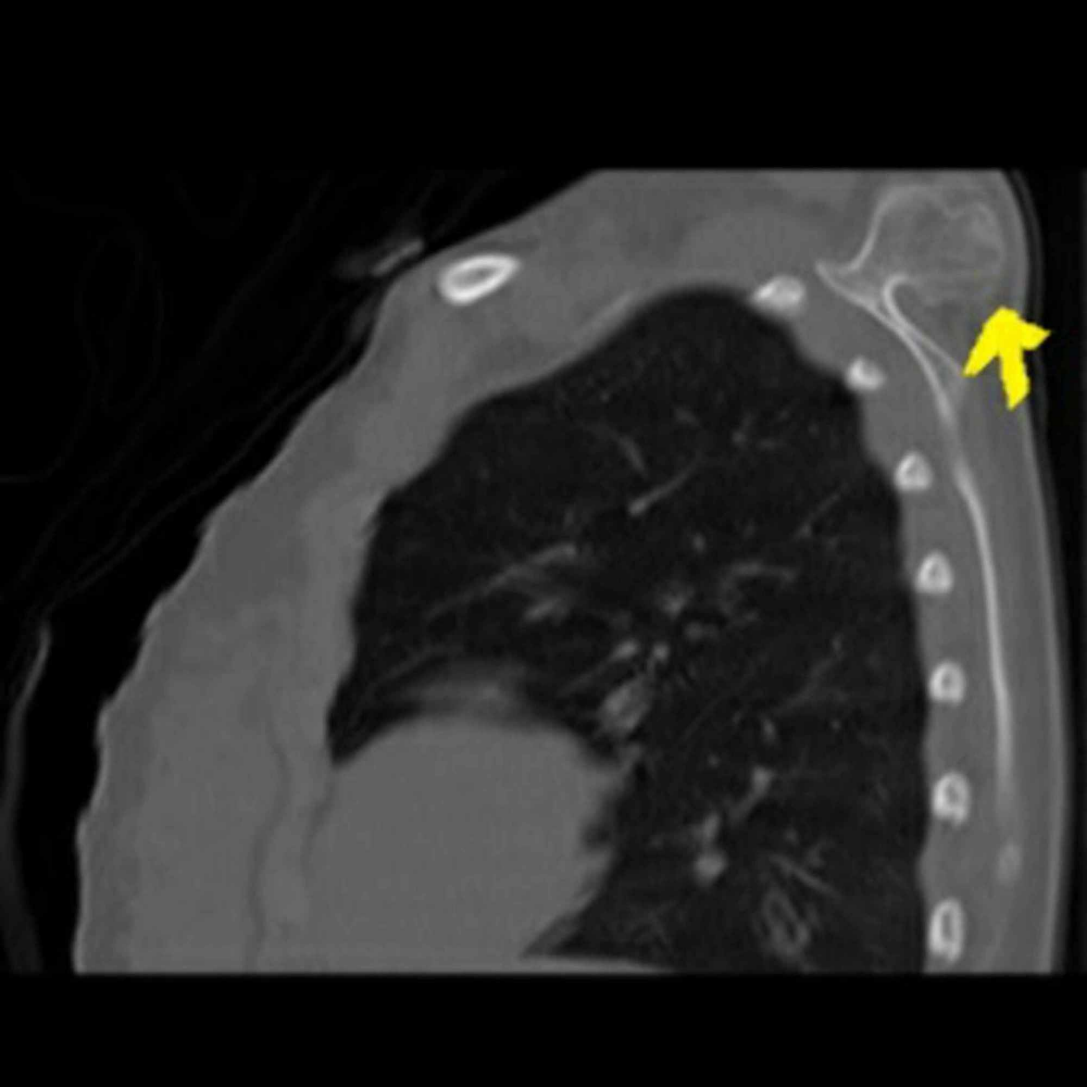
CT detected a pedunculated mass on dorsal aspect of the scapula.
